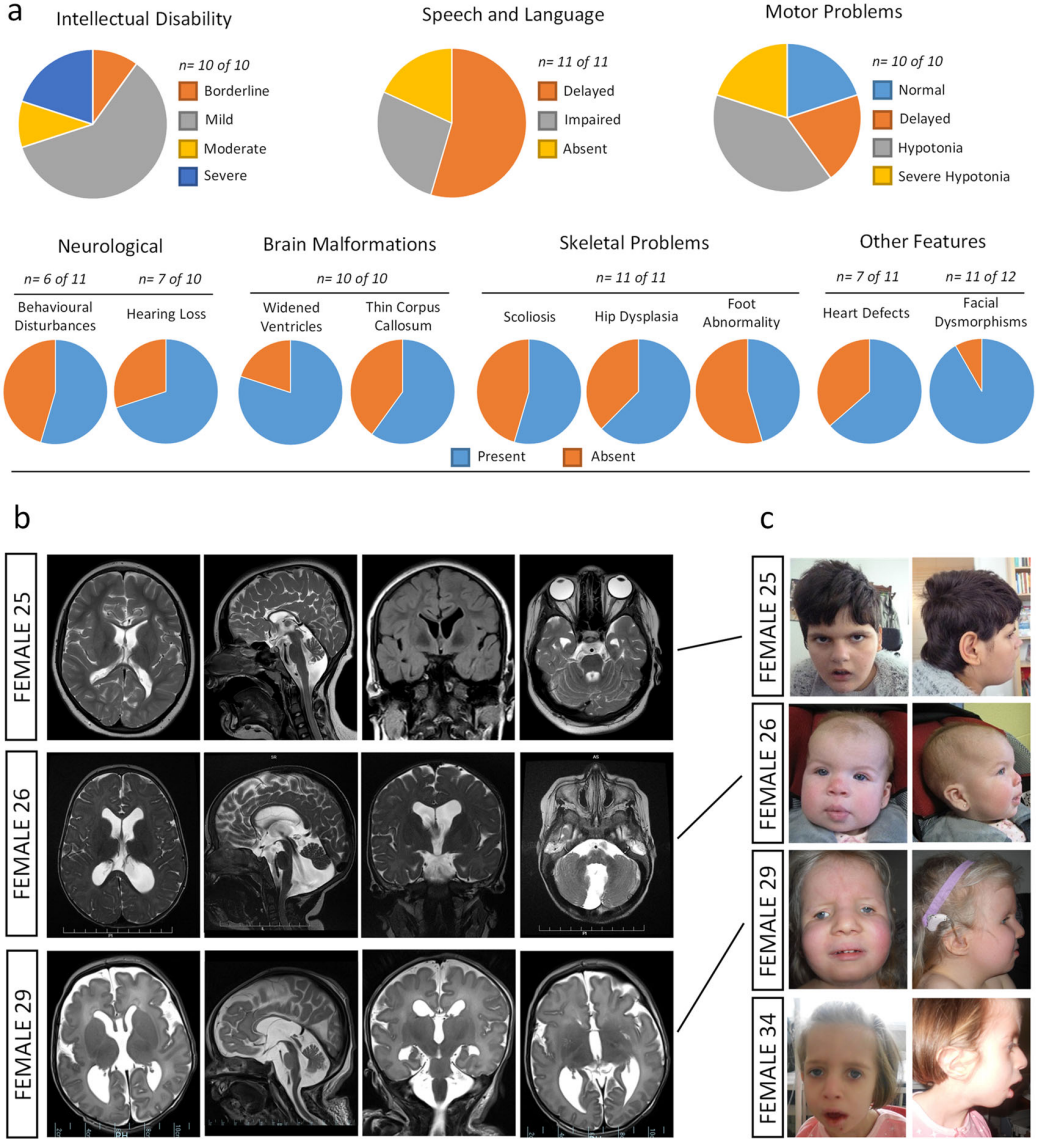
Phenotypic features of females with *USP9X* missense and single amino acid deletion variants. a Constellation and frequency of major clinical features. b Magnetic resonance imaging (MRI) of brains of affected individuals. Note prominent extra-axial spaces in all, hypoplastic corpus callosum in Females 25 and 29, optic nerve atrophy and in Female 25, Dandy Walker malformation and Blake’s pouch cyst in Female 26, and ventriculomegaly of the 3rd and 4th ventricles in Female 29. c Images of affected females showing facial dysmorphisms, with common features including deep-set eyes, telecanthus, blepharophimosis, a broad nasal tip with wide alae and short collumnella, and low set and dysplastic ears. Written consent was obtained for the publication of photographs.
